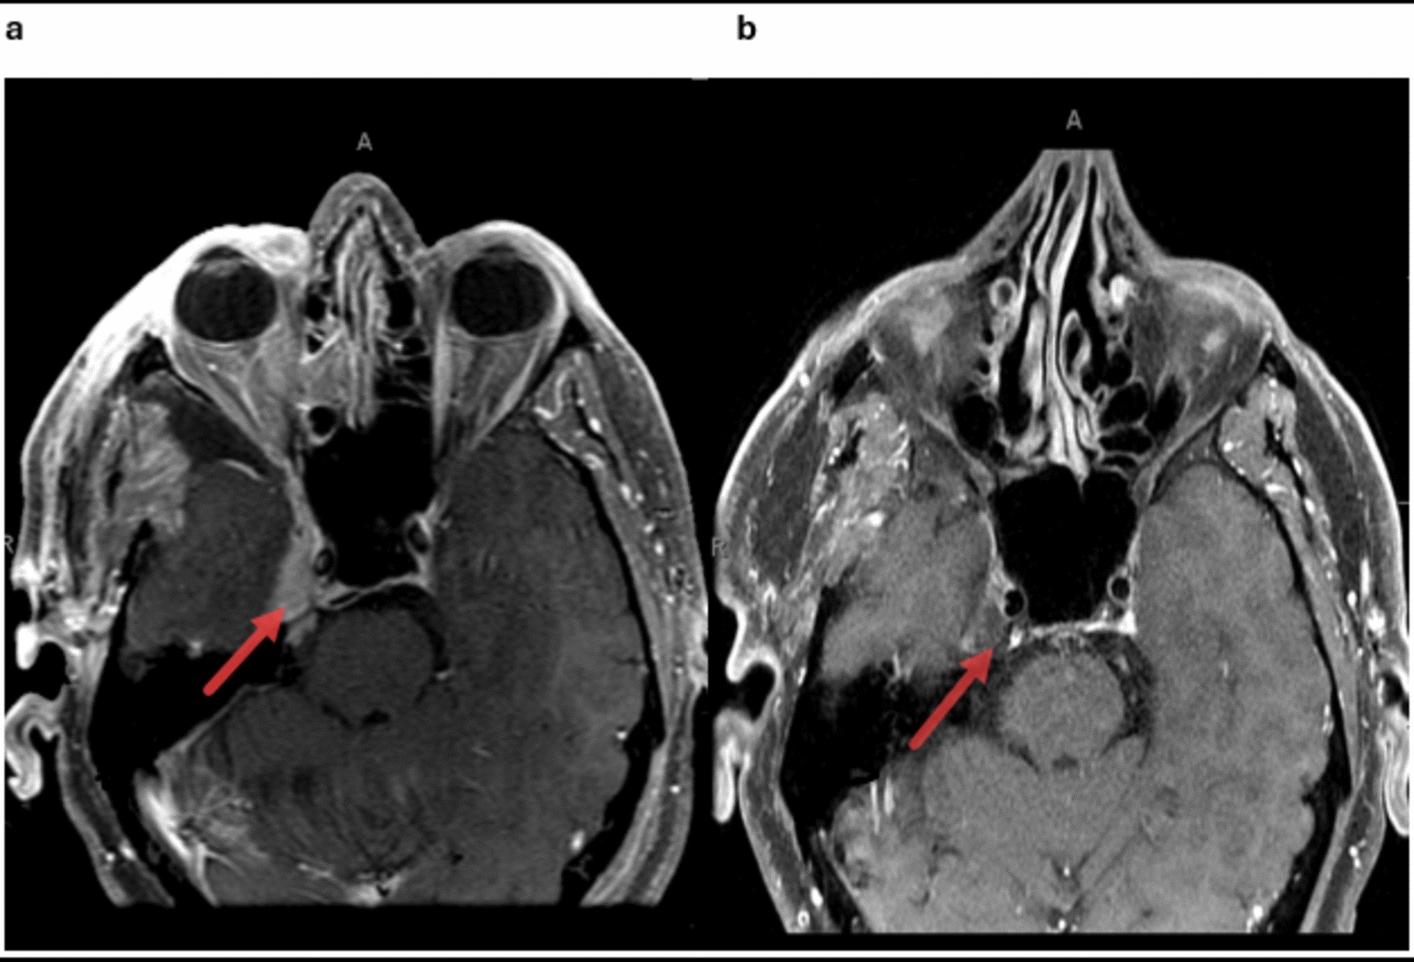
Postoperative and post-treatment brain magnetic resonance imaging with intravenous contrast demonstrates stepwise resolution. Immediate postoperative image (a) showing debulking of the tumor in Meckel’s cave with residual tumor extending posteriorly along the cisternal segment of the trigeminal nerve (red arrow). Additional image from the most recent follow-up (b) showing near enhancement resolution (red arrow)
